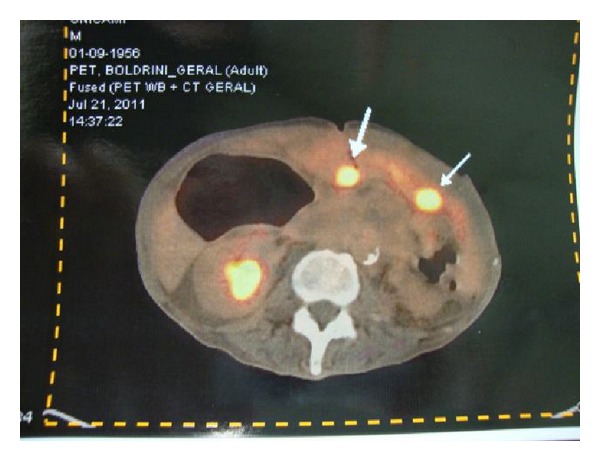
Peritoneal lesions with high SUV on PET-CT.
